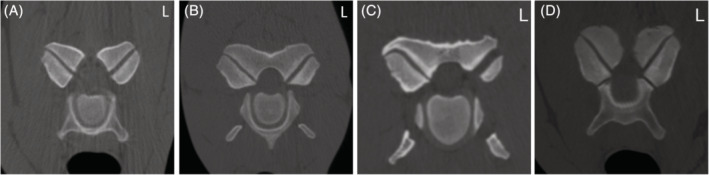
A‐D, Computed tomographic (CT) images depicting grades of degenerative joint disease of the articular process joints. A, Normal (grade 0) articular process joint at C6‐C7 in Case 46; B, grade 1 articular process joint change at C3‐C4 in case 47; C, right sided grade 2 change to the articular process joint of C4‐C5 in case 14; and D, bilateral grade 3 change of the articular process joints with intervertebral foraminae obliteration of C6‐C7 in case 40
